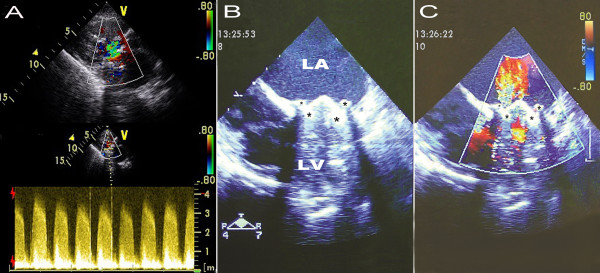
Echocardiography of mitral prosthesis: A) Transthoracic echo reveals immobilization of the mechanical bileaflet with accelerated velocity of transvalvular blood flow; B) and C) Intraoperative transesophageal echo demonstrated prosthetic valve obstruction due to pannus formation (asterisks) on atrioventricular side. LA indicates left atrium; LV indicates left ventricle.
